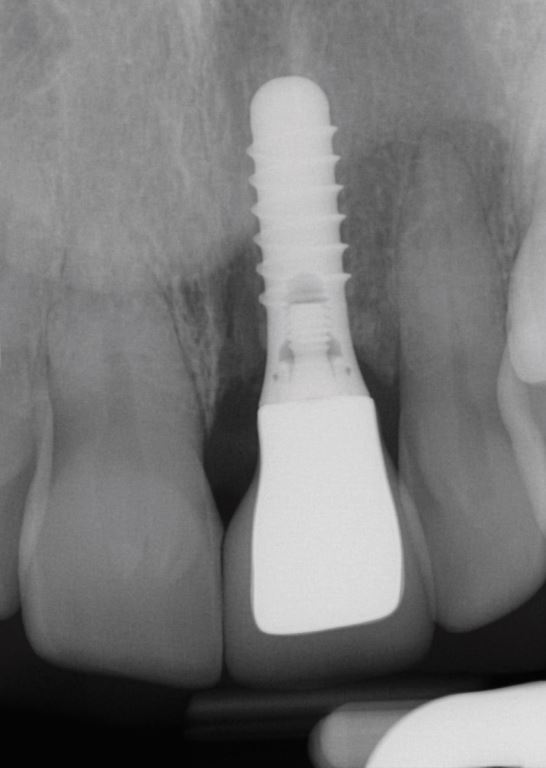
Intraoral periapical radiograph two weeks post debridement showing radiographic bone defect
